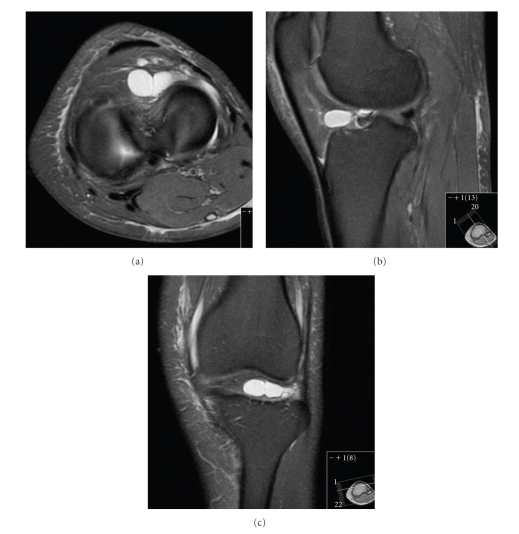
Axial/sagittal/coronal FD FS MRI showing a large multilocular cystic swelling arising from the ACL tibial footprint extending to the infrapatellar fat pad.
